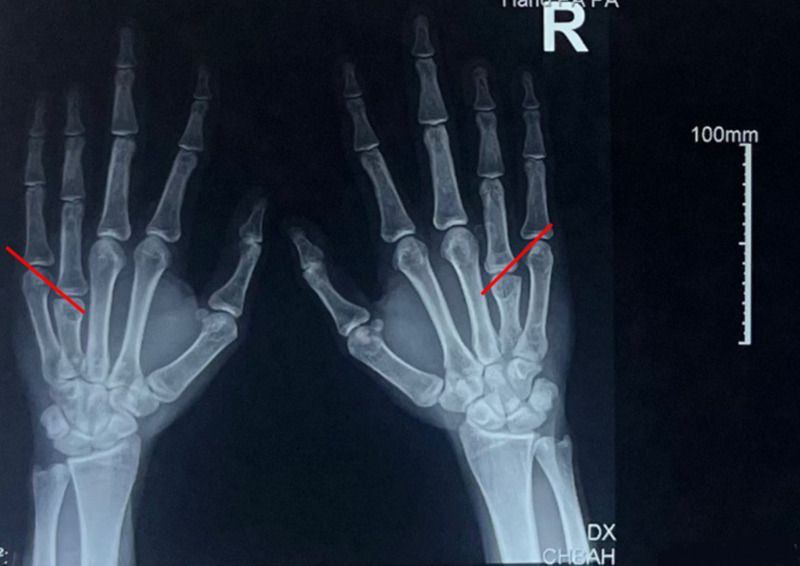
radiograph of hands showing the short 4^th^ metacarpal signs demonstrated by the red lines
